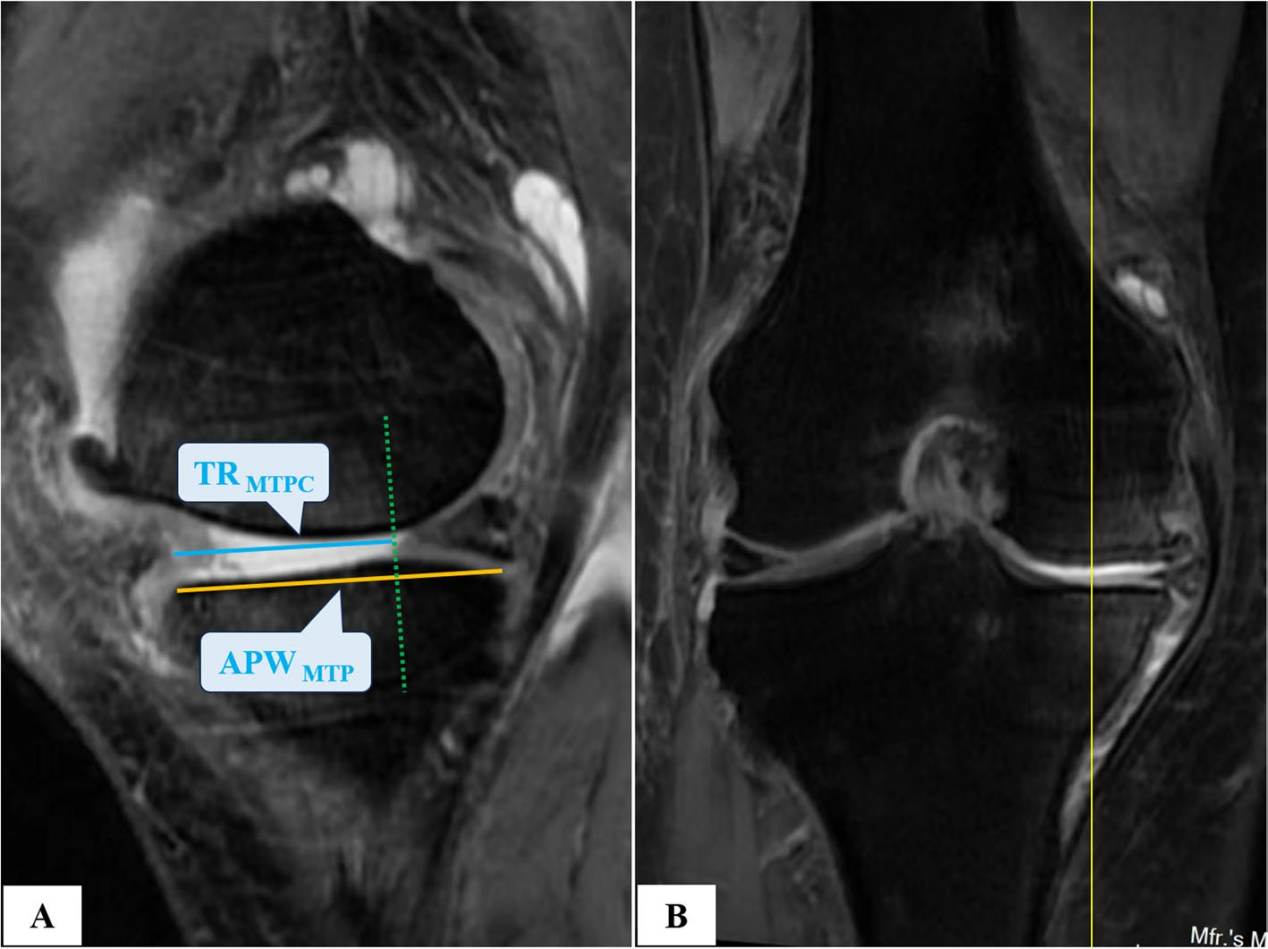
Measurement of parameters on the conventional knee MRI. From the coronal view (B), we selected the sagittal section on which the midpoint of the medial tibial plateau was located (A) to calculate MWPPT%_MRI_ following the measurement of TR_MTPC_ and APW_MTP_ by the same method for calculating MWPPT%_XR_
